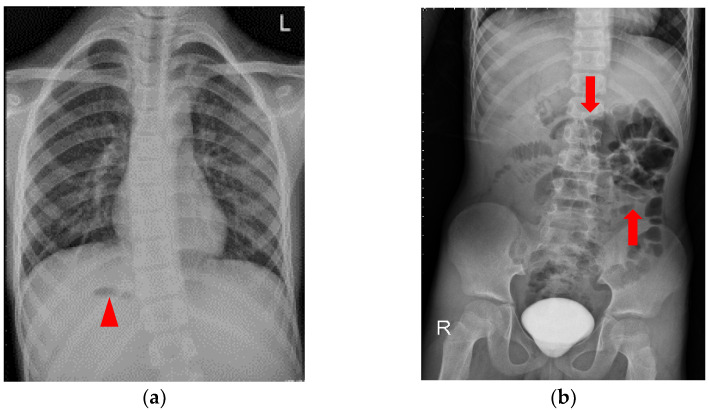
Plain film of the chest and abdomen: (a) right-sided stomach gas (arrow head) and levocardia; (b) midline liver shadow and colon gas on the left side (arrows), suggesting heterotaxy syndrome and malrotation. Contrast medium that had not finished metabolism was retained in the urinary bladder.
